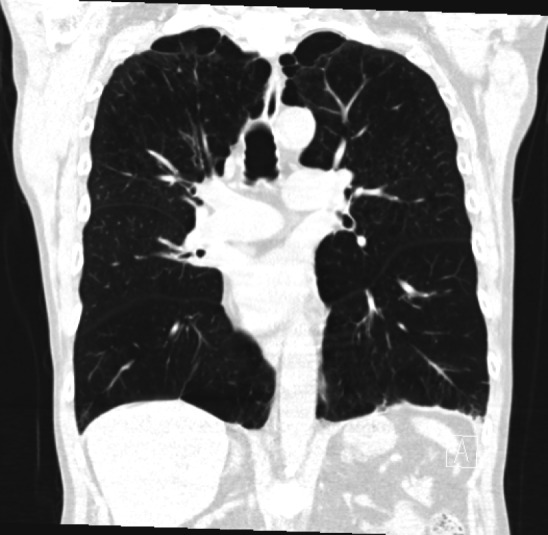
Coronal computed tomography image of the chest showingbiapical subpleural blebs but no identifiable pneumothorax.
